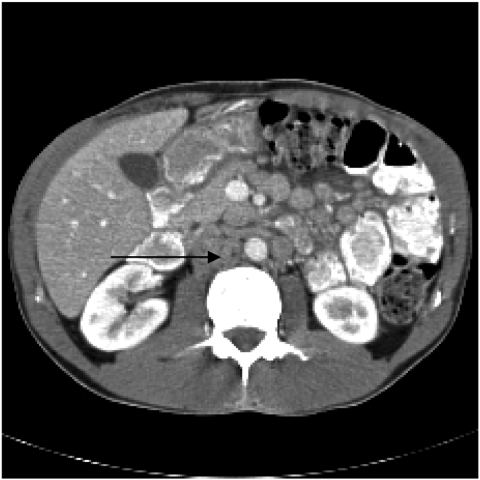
Initial computed tomographic scan of the abdomen, performed after intravenous injection of contrast dye, showing numerous enlarged para-aortic lymph nodes (arrow).
